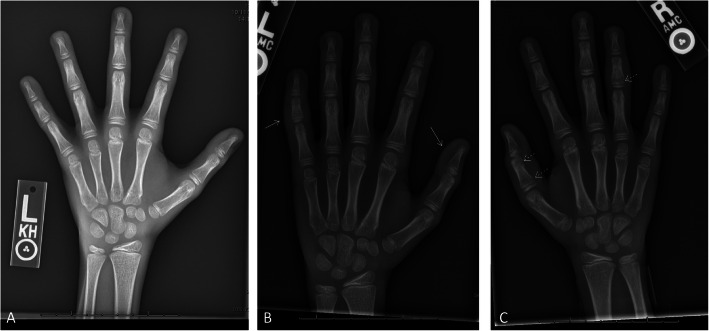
a. Left hand radiograph taken 6 months prior shows delayed bone age, but no other abnormalities of the bones or soft tissues. Shown here for comparison. b. Left hand radiograph at presentation shows soft tissue swelling of the first and fifth digits (solid arrows). c. Right hand radiograph hand shows cortical erosion of the head of the first proximal phalanx and metaphyseal base of the fourth middle phalanx with periosteal reaction and overlying soft tissue swelling (dotted arrows)
